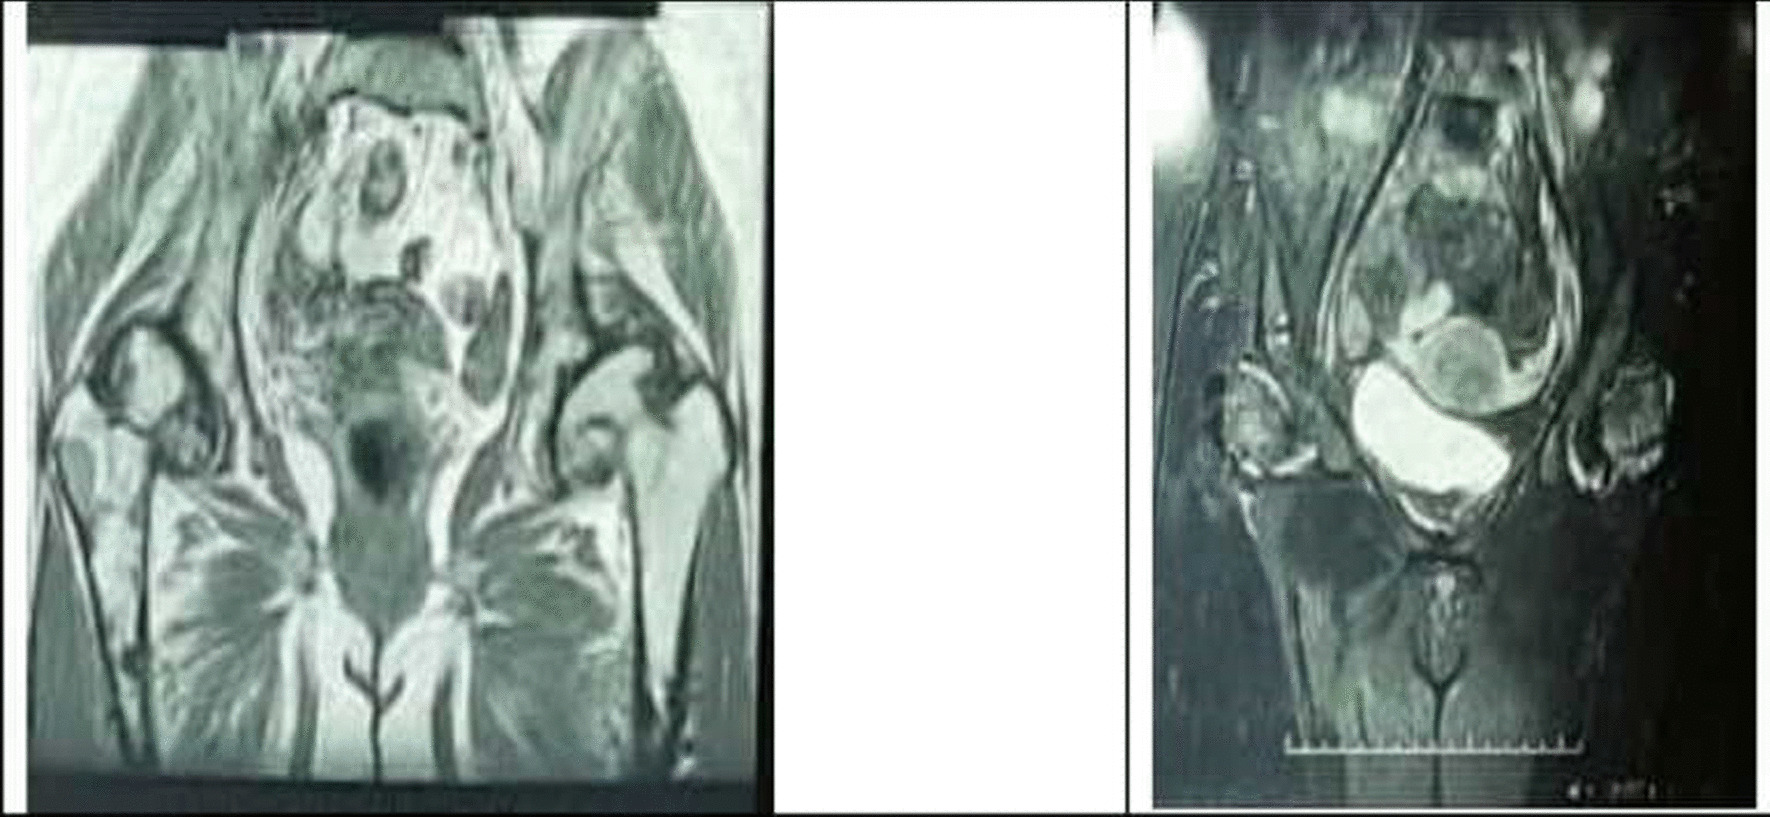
Pelvic MRI of the patient (T1: left, T2: right)
